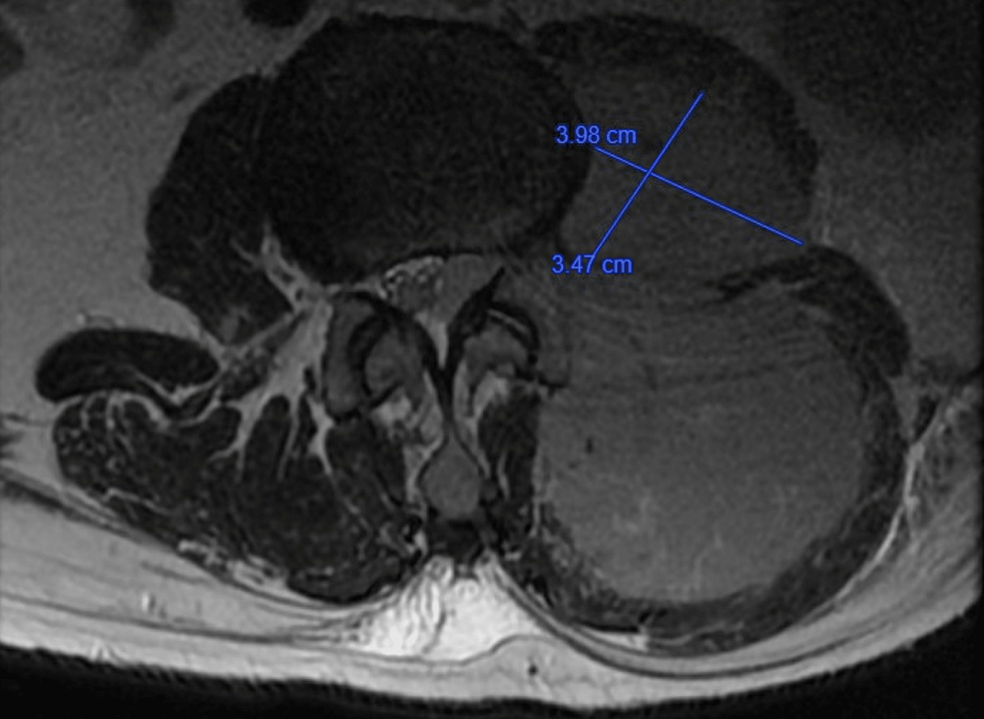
Anteriorly positioned paraspinous element measures 3.98 x 3.47 cm in the T2-weighted axial plane view
